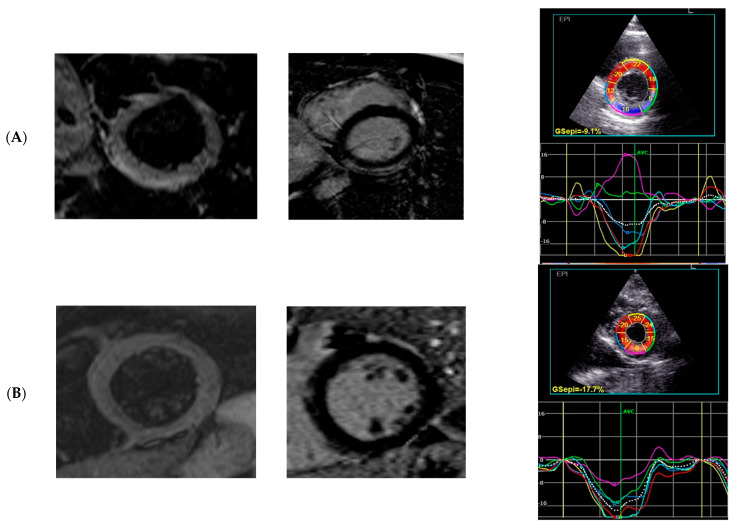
A representative patient with oedema (A) and without oedema (B); basal segments in short axis: CMR—oedema in T2-weighted image, LGE positive in T1 image, 2D STE GCS Epi, respectively.
